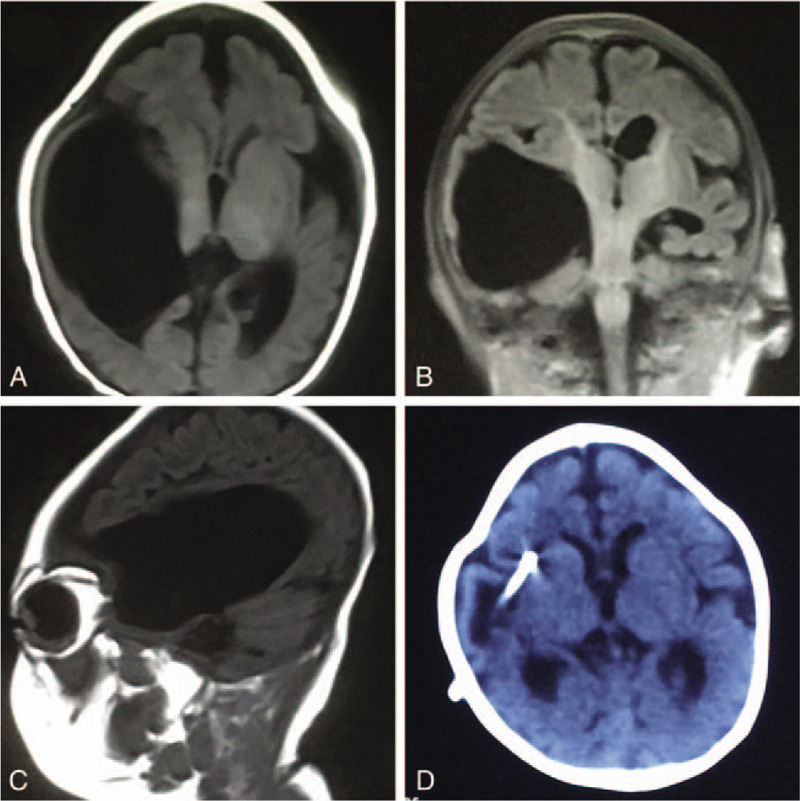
(A–C) Brain magnetic resonance image (MRI) at 4 months after birth. Brain MRI showed a giant cyst in the right lateral ventricle. (D) Head computed tomography (CT) at 6 months after birth: head CT showed the cyst was significantly smaller after 2 months of treatment.
